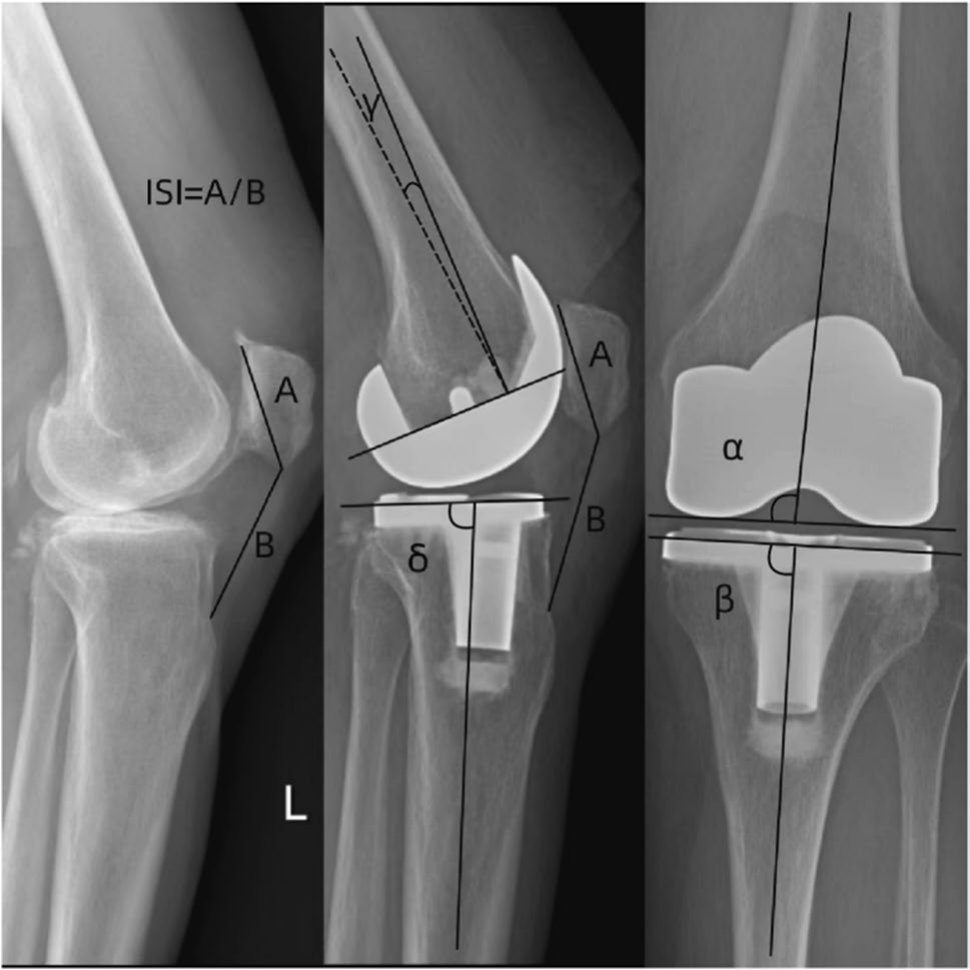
The ISI, *α*-angle, *β*-angle, *γ*-angle, and *ε*-angle were measured on the pre-operative knee lateral X-ray and post-operative knee anteroposterior X-ray and lateral X-ray
